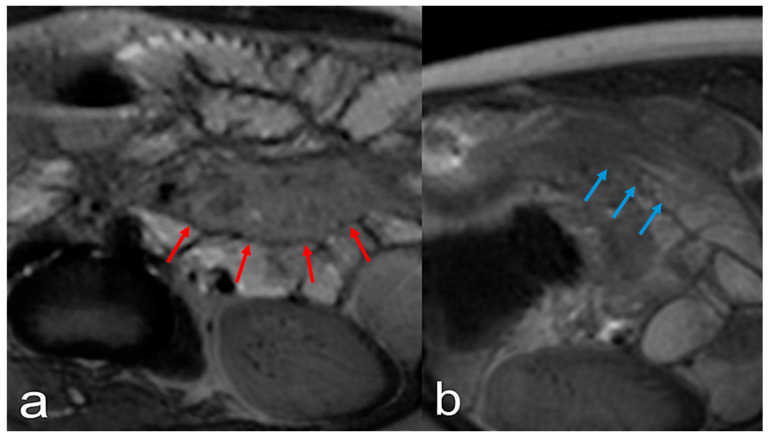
MRI findings in Crohn’s disease: (a) axial MRI showing features of MP, including a pseudocapsule (red arrows), central signal reduction in the surrounding fatty tissue, embedded nodules with a halo sign, and displacement of adjacent bowel loops; (b) axial MRI showing terminal ileitis with marked bowel wall thickening (blue arrows).
